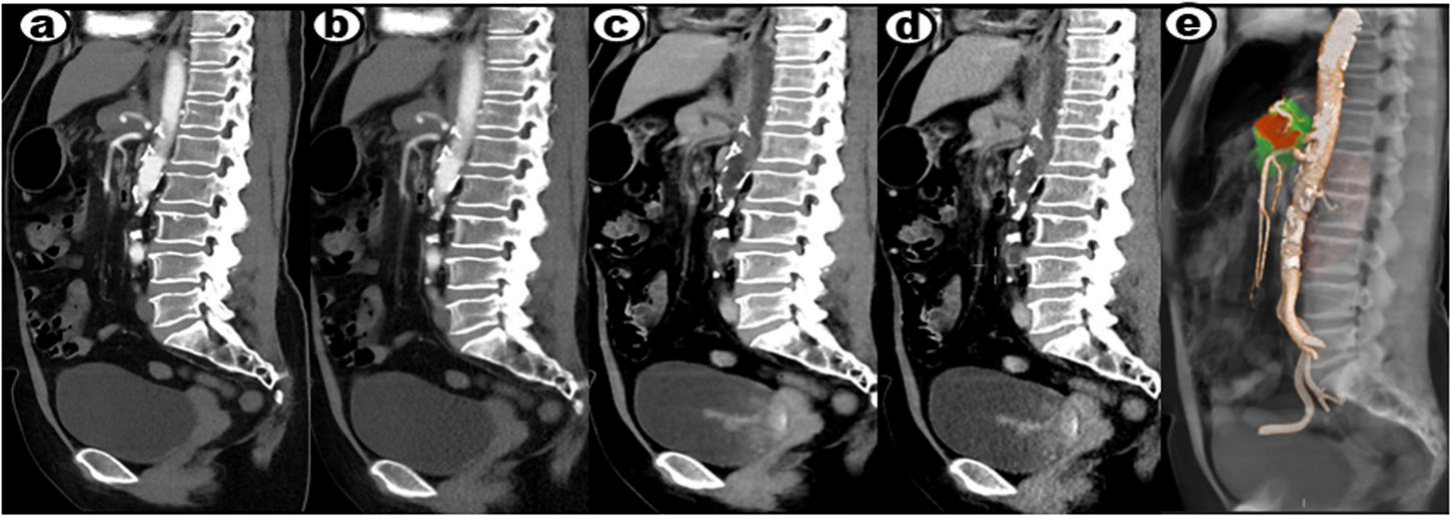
A 56-year-old woman was diagnosed with PDAC involving the body of the pancreas, with invasion of the CA and SMA. a–d Sagittal multi-planar reformation (MPR) CT angiographies were reconstructed using AiCE, AIDR 3D, AiCE–BBCT, and AIDR 3D–BBCT algorithms to visualize the arteries at the same window and center levels. The images allowed for clear visualization of the invaded CA (indicated by an arrow) in each reconstruction. e Additionally, a sagittal GIR fusion image obtained using the AiCE algorithm was generated. In this fusion image, the normal pancreatic parenchyma was represented in green, while the tumor was depicted in red. The invaded CA and SMA were well delineated, providing a comprehensive visualization of the tumor’s involvement with these vessels. The images were acquired with a slice thickness of 1 mm. The BBCT technique effectively reduced the enhanced CT value within the vascular lumen, resulting in better visualization of the vascular wall
